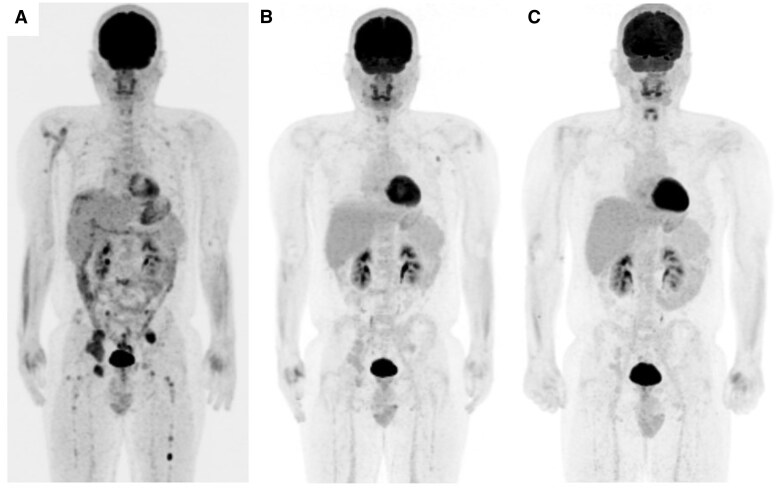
PET/CT images of the patient. (A) Baseline before alectinib treatment, (B) three months of alectinib, and (C) one year of alectinib.
